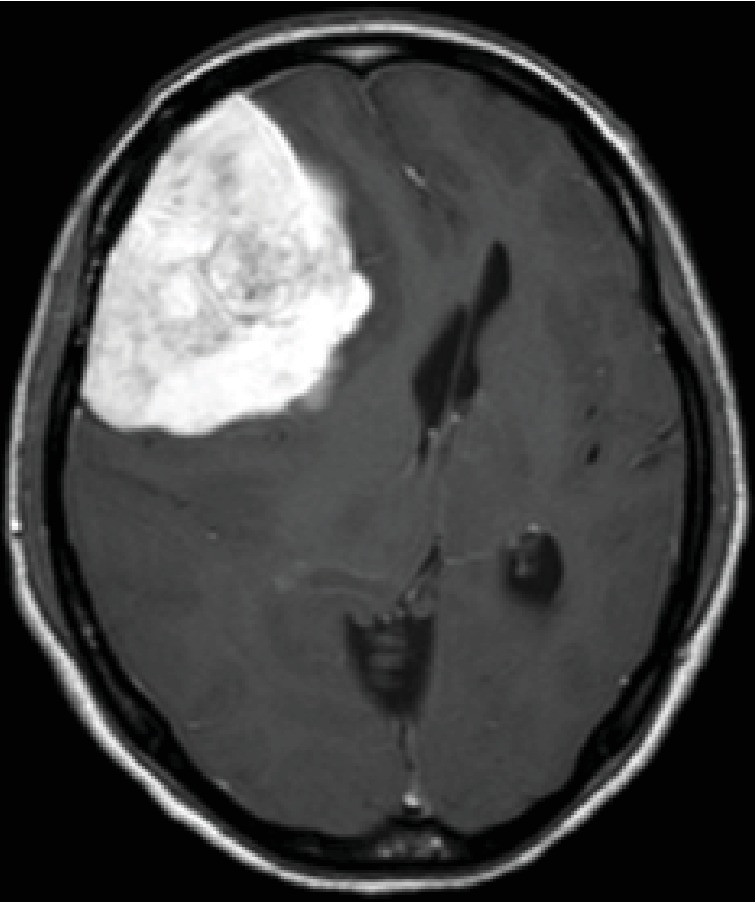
Gadolinium- (Gd-) based contrast-enhanced MRI significantly enhanced the signals.
